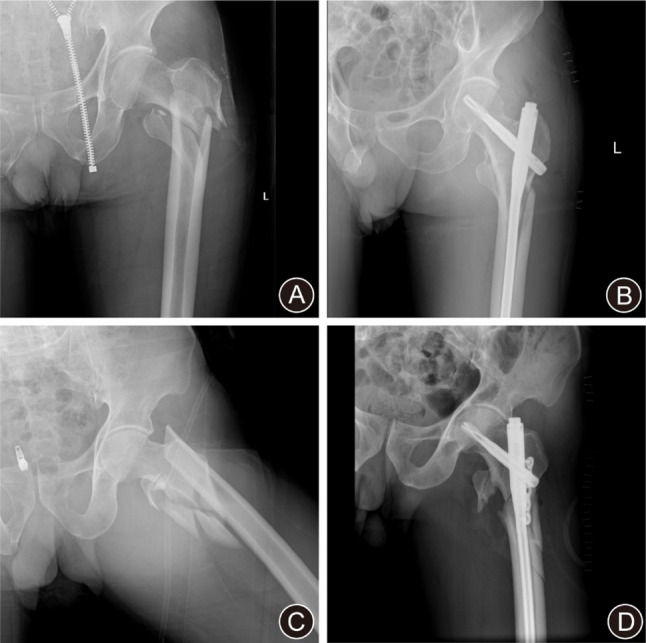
Representative cases. A 72-year-old male patient presented with left hip pain and limited range of motion after a fall. Figure A shows the preoperative anteroposterior radiograph demonstrating a left subtrochanteric femoral fracture (Seinsheimer type IV). Figure B shows the postoperative anteroposterior radiograph revealing satisfactory positioning of the intramedullary nail fixation, with the cephalomedullary screw centrally placed within the femoral head and neck, adequate restoration of the femoral neck-shaft angle, and the lesser trochanter fragment left unreduced and unfixed. A 57-year-old male patient presented with left hip pain and restricted range of motion following a fall injury. Figure C shows the preoperative anteroposterior radiograph demonstrating a left subtrochanteric femoral fracture (Seinsheimer type IV). Figure D shows the postoperative anteroposterior radiograph revealing satisfactory fracture reduction with well-positioned internal fixation implants
